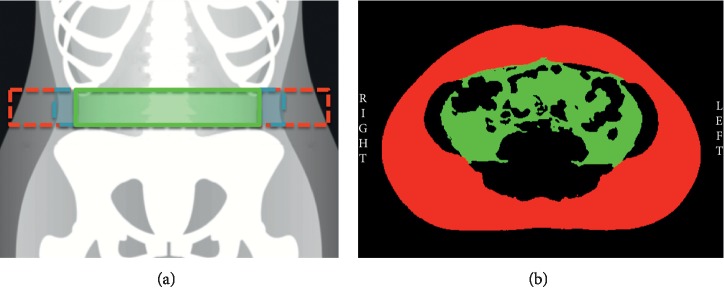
Subcutaneous (red) and visceral (green) adipose tissue compartments shown on DXA (a) and MRI (b). Blue dashed lines on DXA panel show the abdominal wall musculature.
